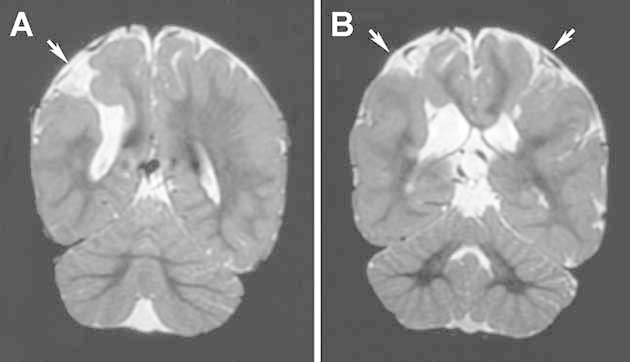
Coronal T2 MRI imaging from two patients demonstrates unilateral, right-sided open lip schizencephaly (A) and bilateral schizencephaly with closed lip on the right and open lip on the left (B) (arrows).
